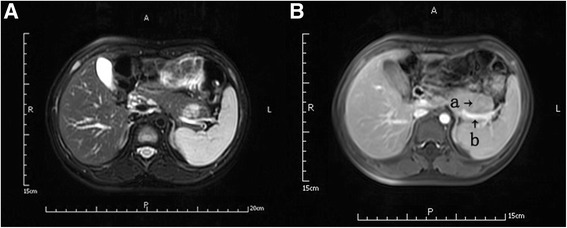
Preoperative abdominal dynamic contrast-enhanced MRI showing tumor at pancreas tail. A T2-weighted MR image showing loss of the normal shape of the pancreas tail and the clear margin of the tumor. B The nutrient vessel stems from the spleen vein. a nutrient vessel; b spleen vein
